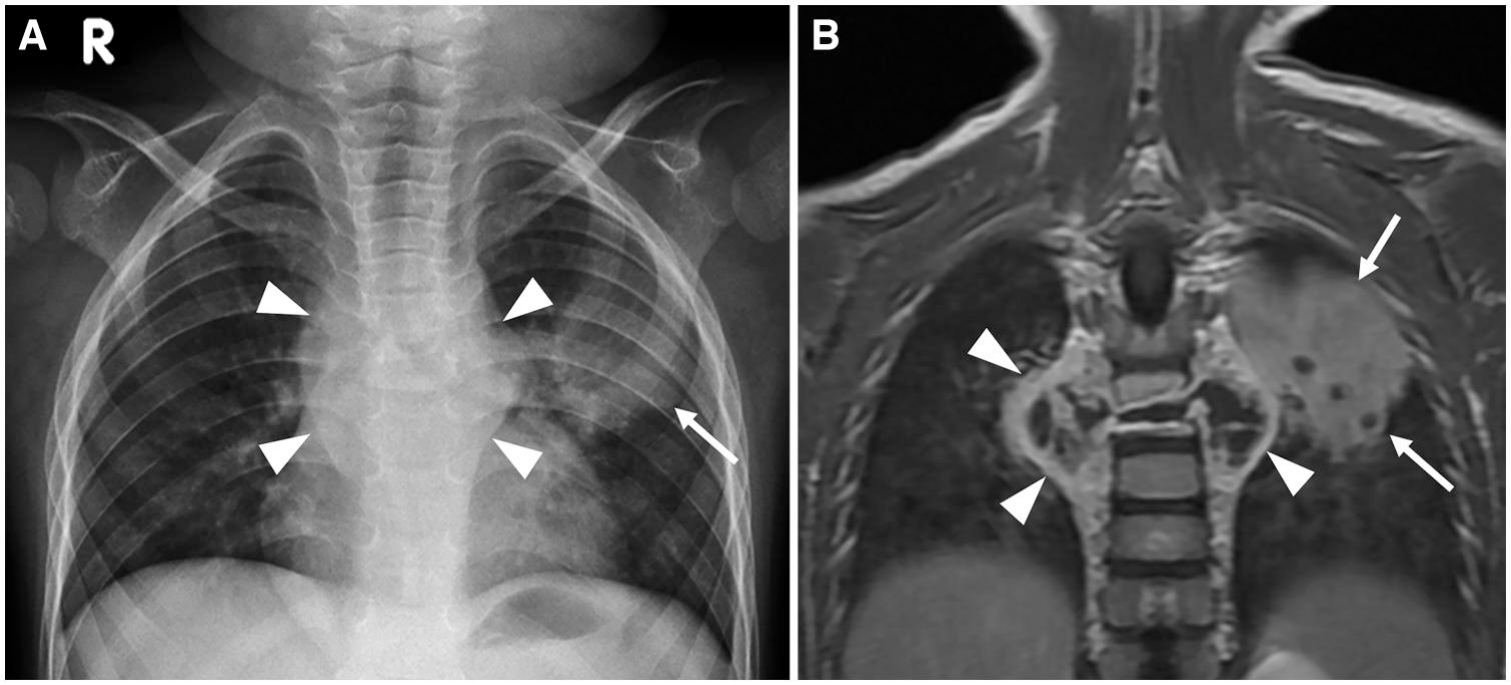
Primary pulmonary TB and thoracic spine TB in a 3-year-old girl. (A) Frontal chest radiograph shows patchy infiltration in the left upper lobe (white arrow) and paravertebral soft tissue swelling (white arrowheads). (B) Coronal contrast-enhanced T1-W MRI shows consolidation of the left upper lobe (white arrows) and destruction of the thoracic vertebrae with paravertebral abscesses (white arrowheads).
